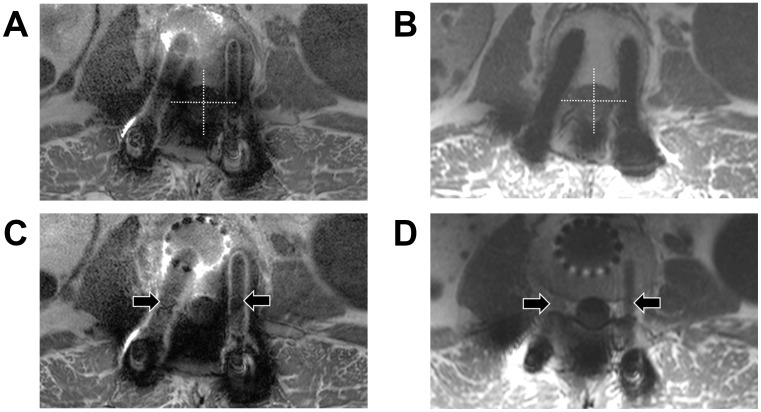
Visibility score of central canal and nerve roots in epidural space in axial images. These four axial images are obtained from a 68 year old male who underwent inter-body fusion at L4-5 and posterior instrumentation at L2-L5 level due to loosening of pedicle screws and infection of previous metallic implants which were inserted for L4 compression fracture. The time interval between surgery and the spine MRI was 5 years and 10 months. Axial fast spin echo T1-weighted image after administration of contrast media (a) shows grade 0,1,0,0 (observer 1) and 0,0,0,0 (observer 2) of visibility of each quadrant of central canal at L3 level. (Right upper, left upper, right lower and left lower, respectively) FSE-SEMAC T1- weighted axial image after administration (b) of contrast media demonstrated grade 2,2,1,2 (observer 1) and grade 2,1,2,1 (observer 2) of each quadrant of central canal. Axial fast spin echo T1-weighted image after administration of contrast media (c) shows grade 0,0 (observer 1) and grade 1,1 (observer 2) in right and left nerve root (arrows), respectively. After application of SEAMC technique, Axial FSE-SEMAC T1- weighted image after administration of contrast media (d) demonstrated grade 3,2 (observer 1) and grade 2,2 (observer 2) in right and left nerve root (arrows), respectively.
